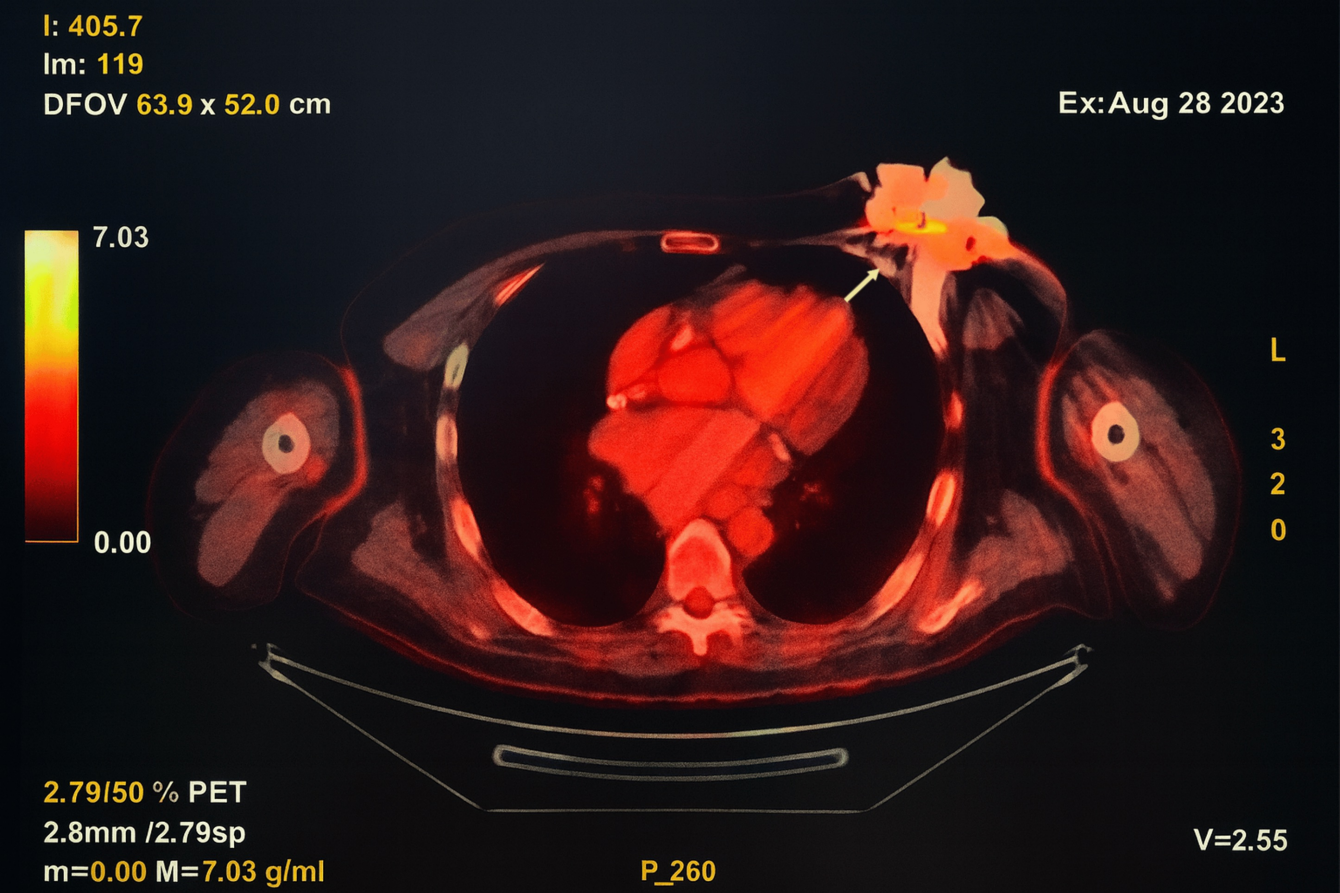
18F-FDG PET-CT showing intense hypermetabolism around the defibrillator
